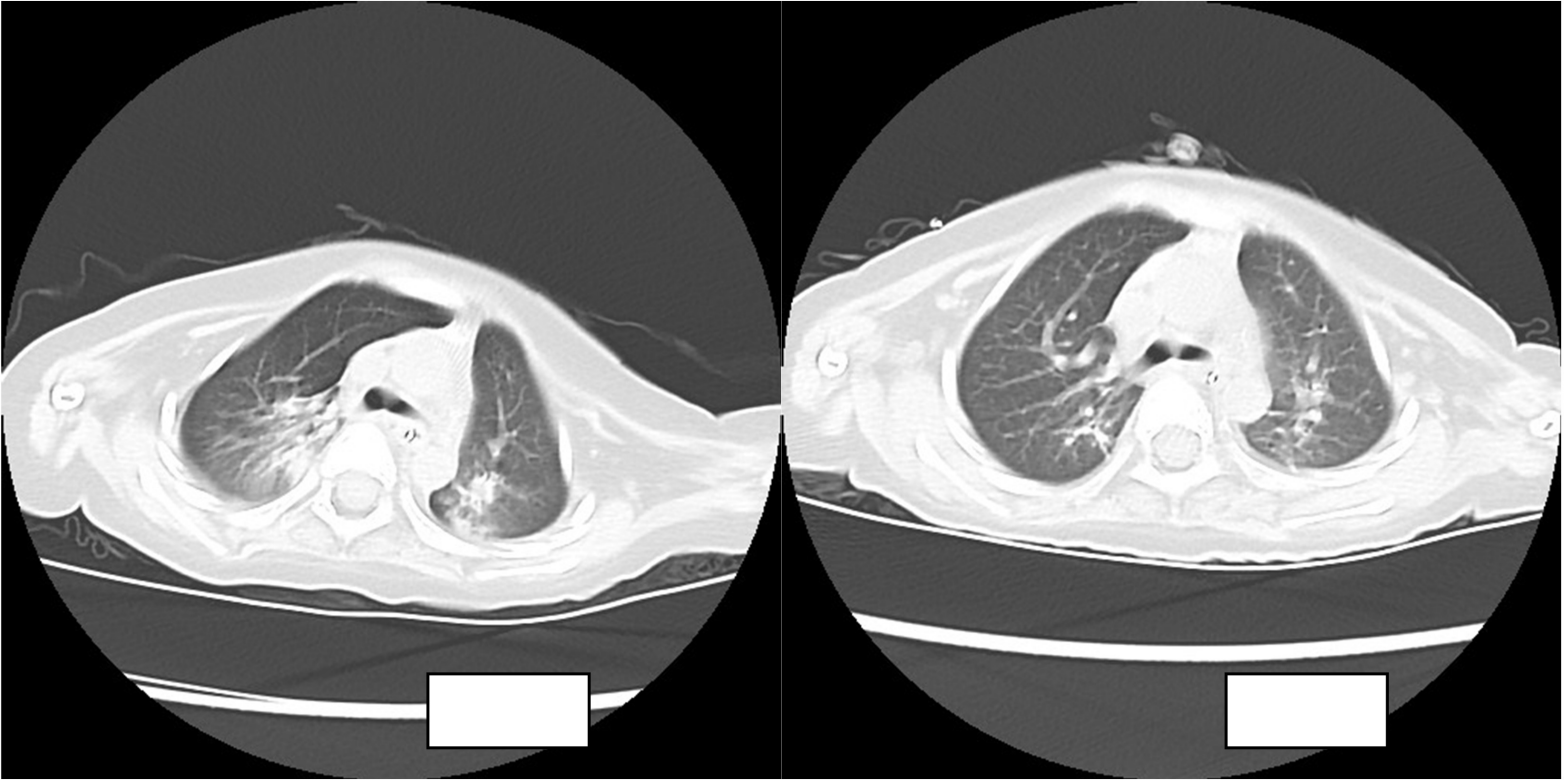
Comparison of the patient’s chest CT before and after treatment.
